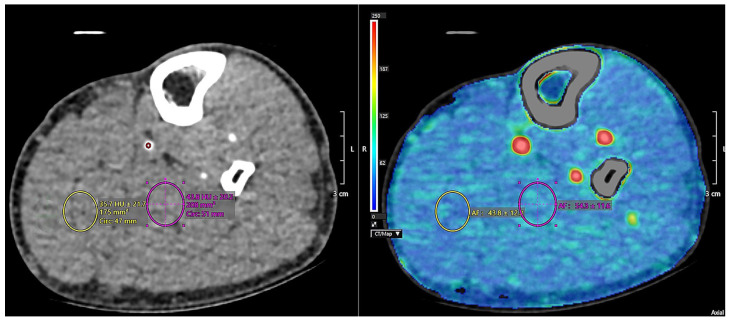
Perfusion computed tomography section.
